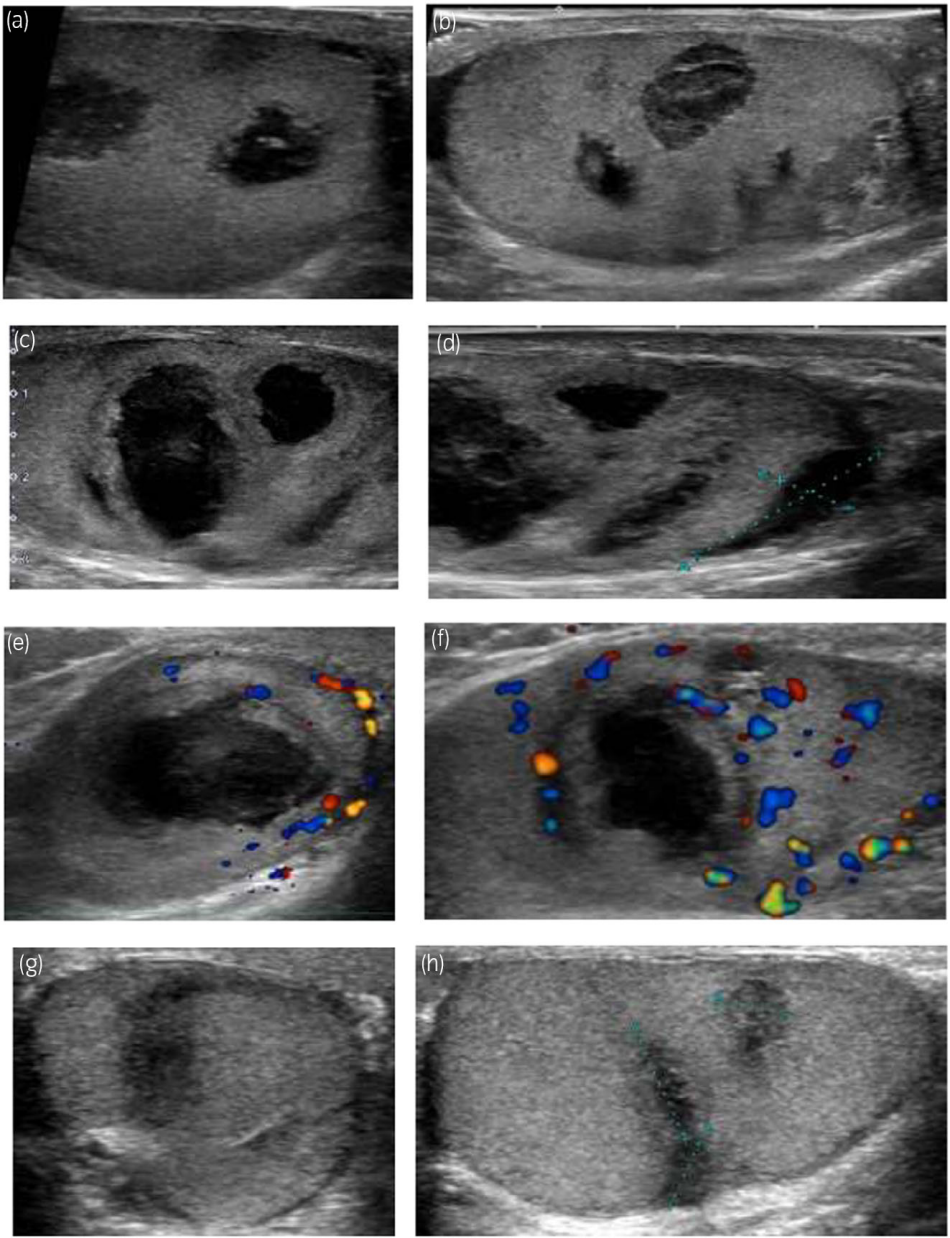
The time‐elapse ultrasonography images of the right testis. Nodules disappeared 6 months after the first visit. The left‐side images of each line are transverse sections, and the right‐side images are sagittal sections. (a,b) Day 1; (c,d) Day 5; (e,f) Day 13; (g,h) Day 182.
